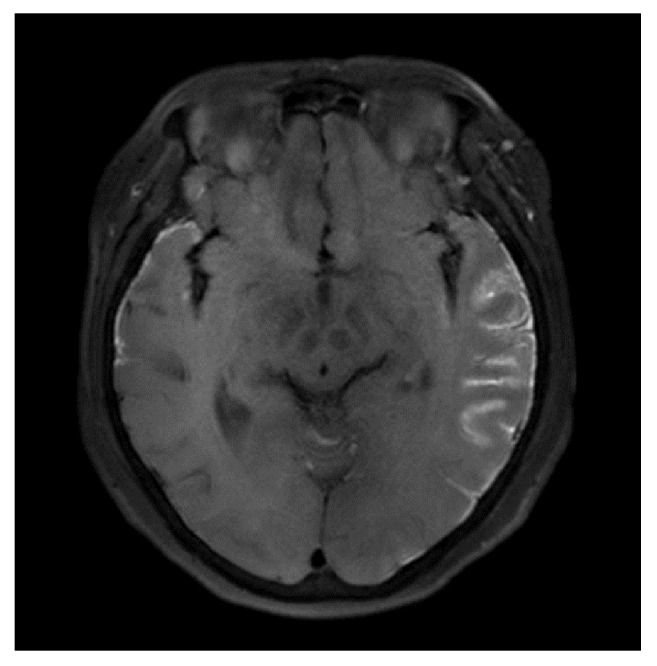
Cranial-enhanced MRI T1-weighted images reveal abnormal linear hyperintensity mainly on the left lateral lobe.
